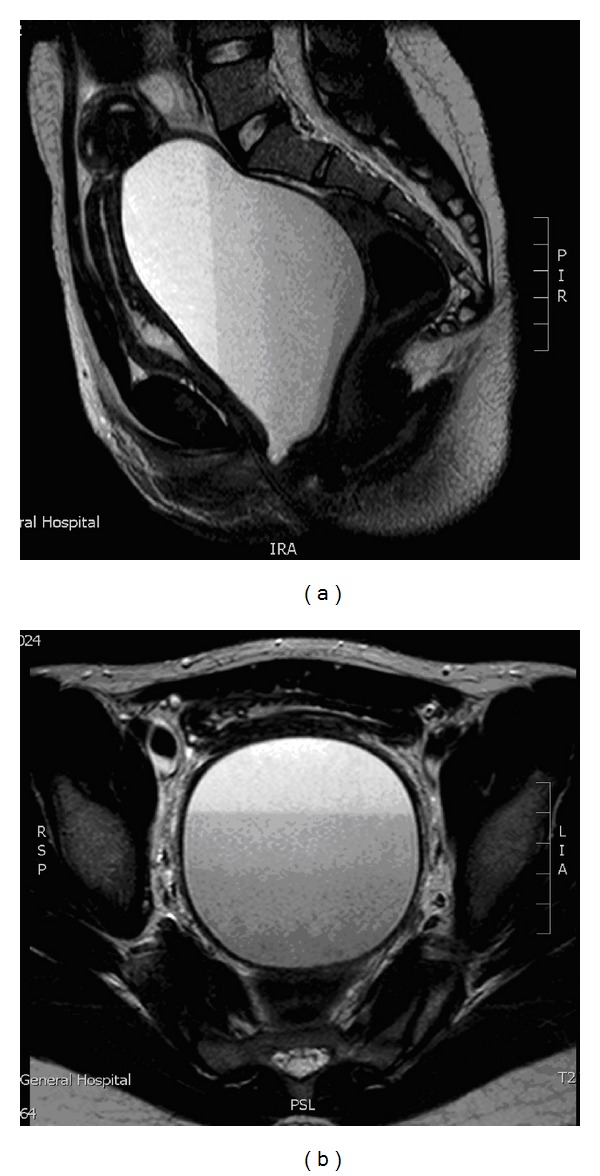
MRI scans showing the differential layers of fluid indicative of blood products.
